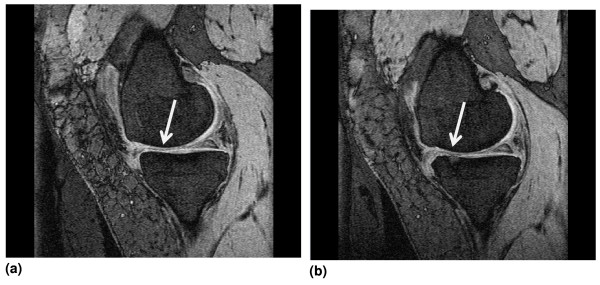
Magnetic resonance images. (a) Magnetic resonance image of knee showing no bone marrow lesion (BML) and a grade 2 medial tibial defect at baseline. (b) Magnetic resonance image showing an incident medial tibial BML and a grade 3 medial tibial defect above the BML at follow up.
